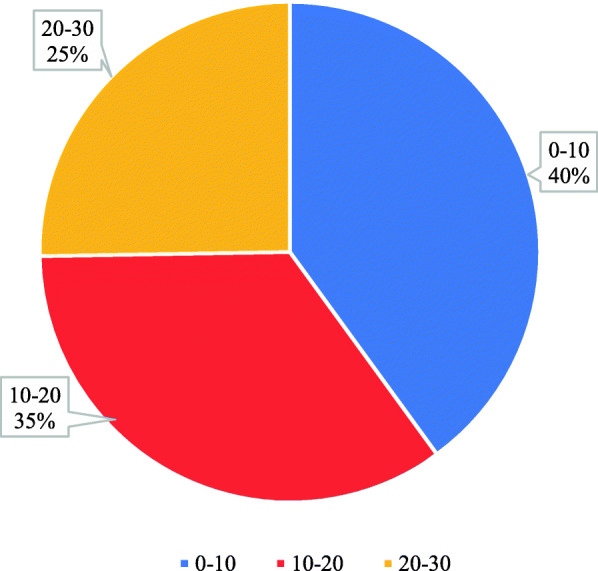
The average number of CT scan cases per duty witnessed by the participants
